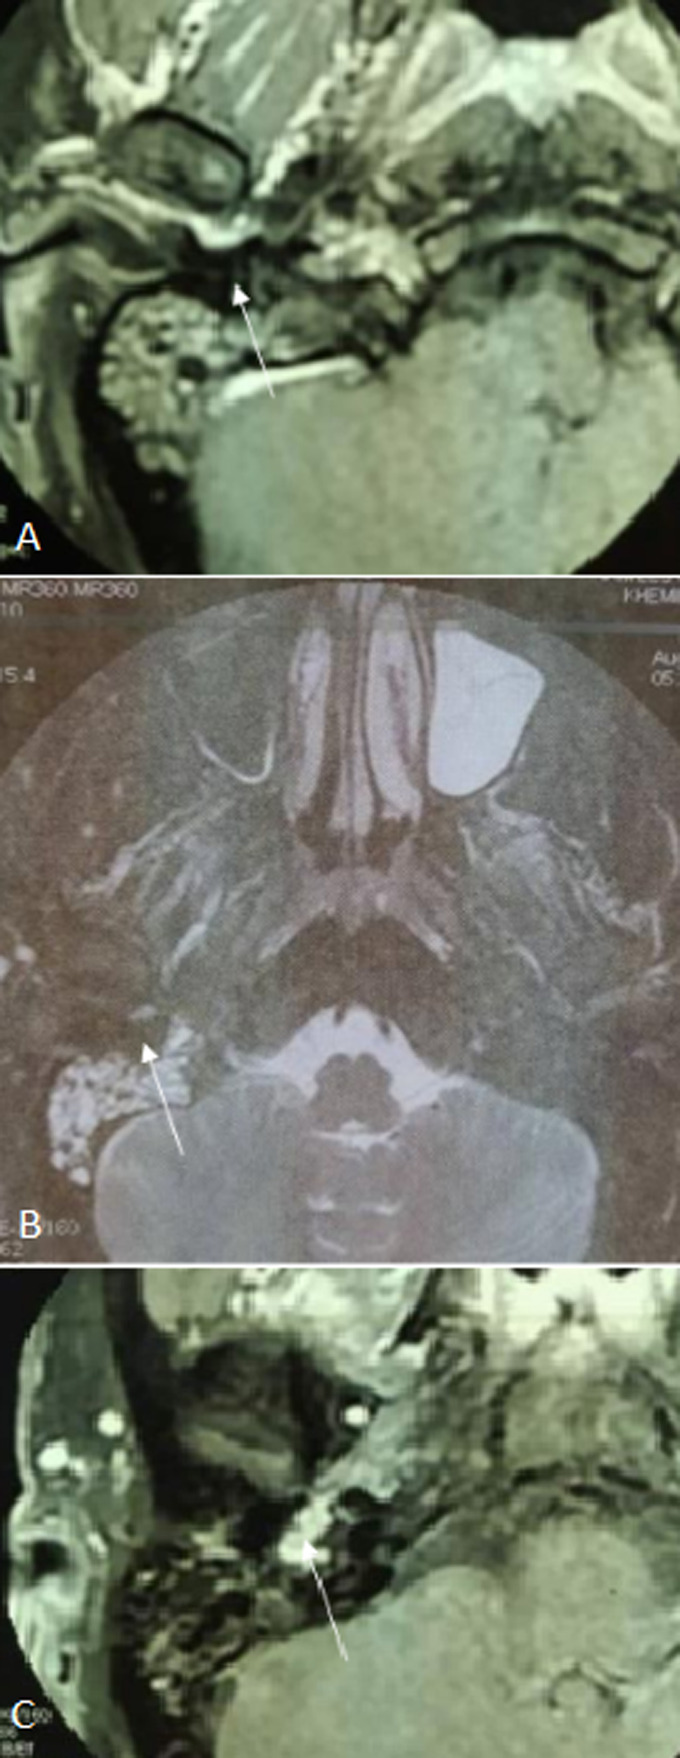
MRI showing a 15 mm mass of the right tympanic cavity, with a “salt and pepper” pattern of hypo intensity and hyper intensity on T1-weighted images (A), relatively higher in intensity on T2-weighted images (B), and strongly enhanced after gadolinium injection (C)
